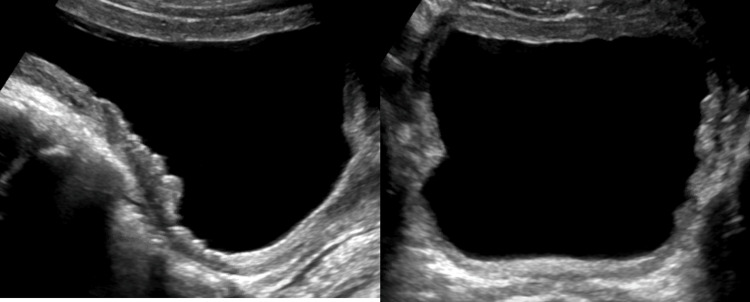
Grayscale ultrasound images of the urinary bladder. Sonographic evaluation of the Urinary bladder demonstrates a thickened and trabeculated urinary bladder wall.
